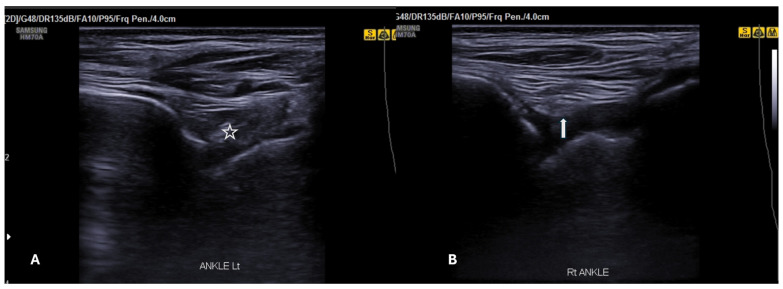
Example of point-of-care ultrasound with HEAD-US scoring system in a patient 17 years old with severe haemophilia A: Ventral longitudinal image plane of both the ankle joints, over the anterior tibiotalar recess. (A) Severe synovitis (star) fills the whole, of the recess volume (synovitis score 2). (B) Hypertrophic synovium (arrow) fills < 50%, of the recess volume (synovitis score 1).
